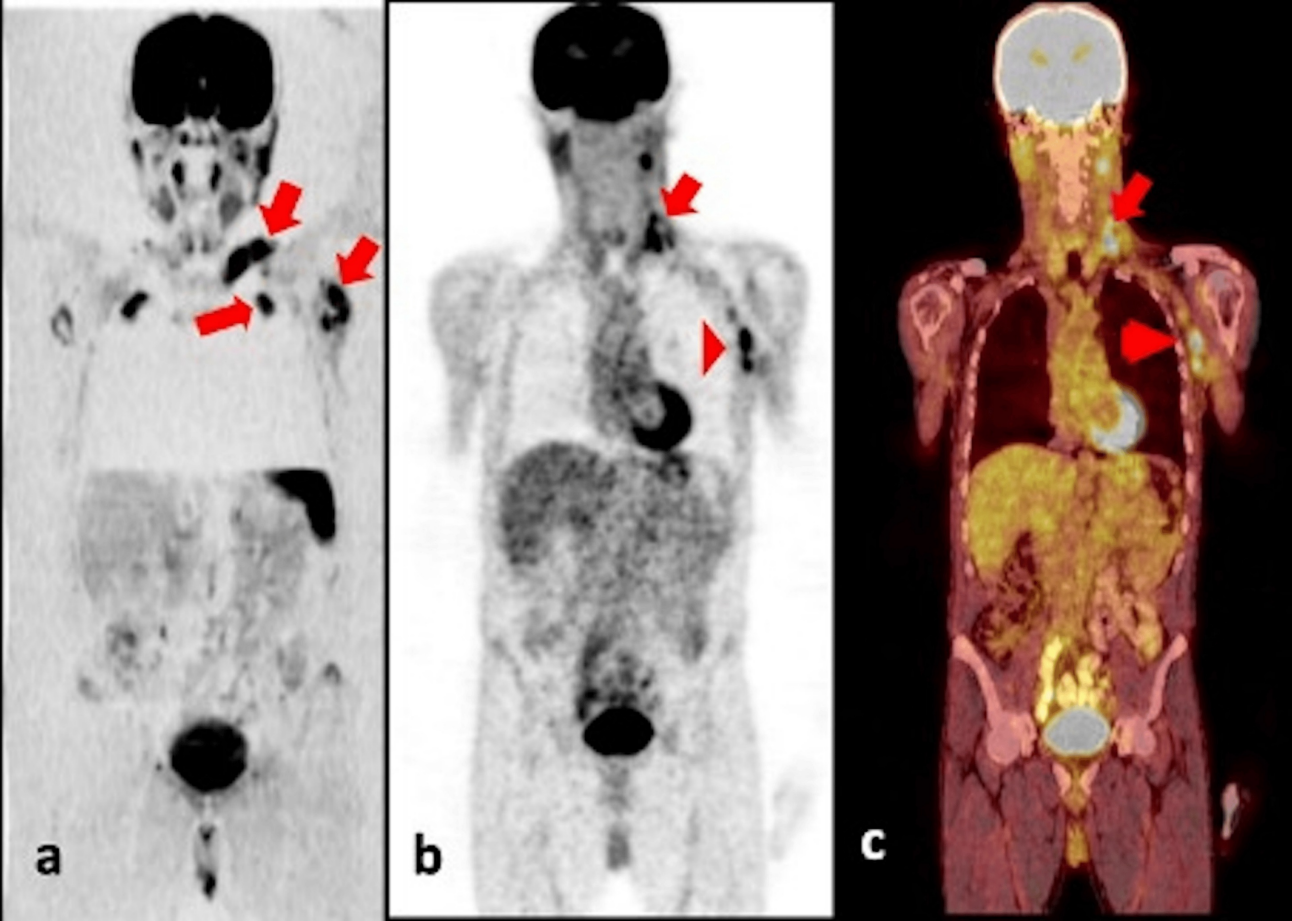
A 62-year-old male patient diagnosed with parotid gland adenocarcinoma. (a) Coronal inverted whole-body DWI examination shows enlarged lymph nodes with pathological signal changes in the left supraclavicular and axillary regions. (b, c) PET and fused PET/CT images reveal increased metabolic activity in the lymph nodes in the left supraclavicular and axillary regions. DWI: diffusion-weighted imaging, PET/CT: positron emission tomography/computed tomography.
